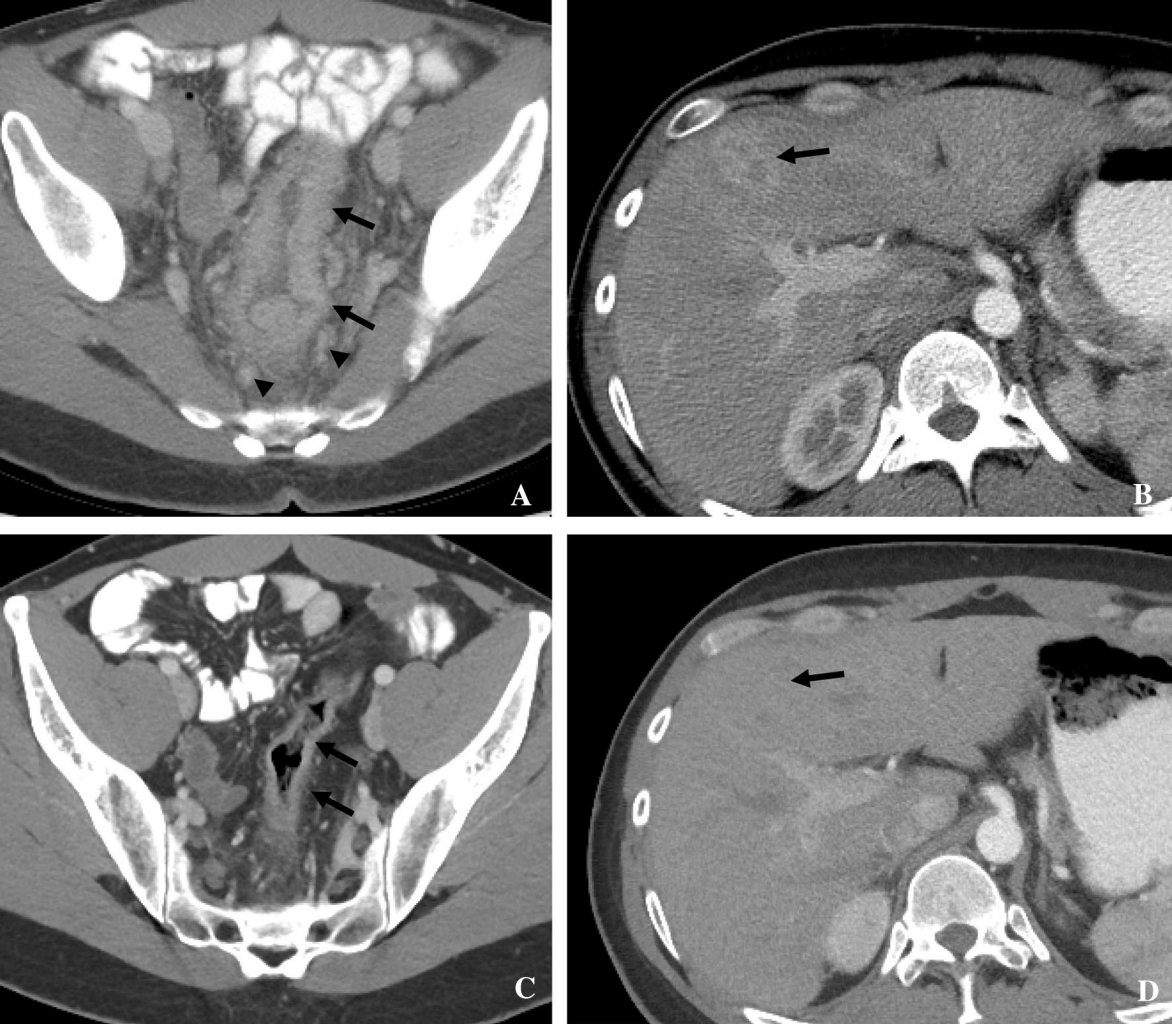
32-year-old male with HIV and KS. Contrast-enhanced CT showing A long segment thickening of the recto-sigmoid colon (arrows), enhancing perirectal lymph nodes (arrowheads), and B enhancing liver lesion secondary to KS, which resolved post-chemotherapy C, D
